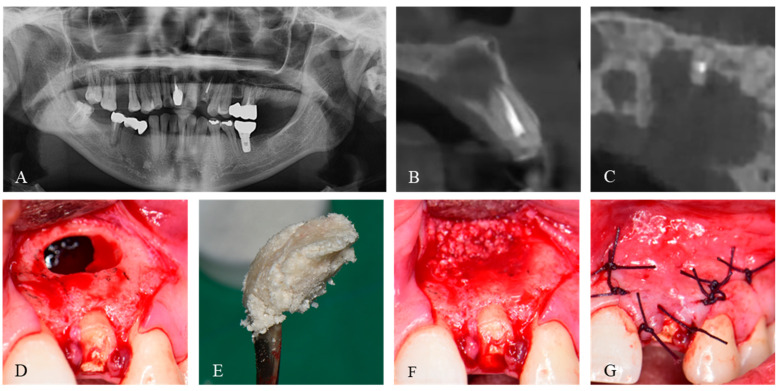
Pre- and intra-operative images. Pre-operative radiographs showed a cystic lesion on the left anterior maxilla and dehiscence of the palatal bone. (A) Panoramic X-ray, (B) Sagittal section of CBCT, (C) Axial section of CBCT. (D–G) Intra-operative images. (D) Bone defect with bucco-palatal bony dehiscence after the enucleation of cyst and apicoectomy procedure on the left maxillary lateral incisor. (E) After hydration of M-DDM according to the manufacturer’s instruction, M-DDM obtained a high viscosity that could mold its shape. (F) M-DDM was grafted into the cystic defect. (G) Primary closure with 4-0 vicryl.
